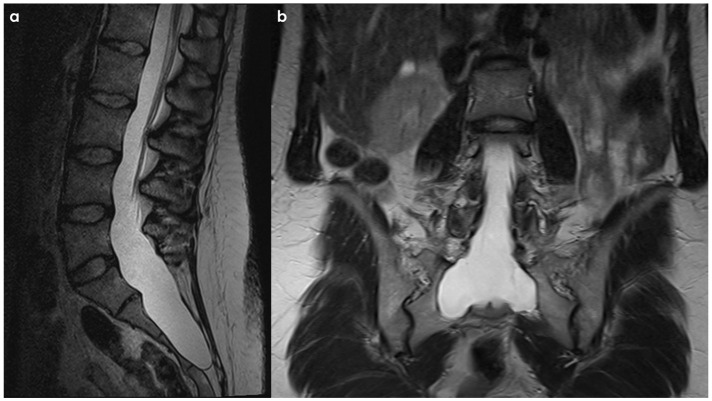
Dural ectasia with megasac in lumbosacral MRI (T2W) in sagittal (a) and coronal reconstructed plane (b).
